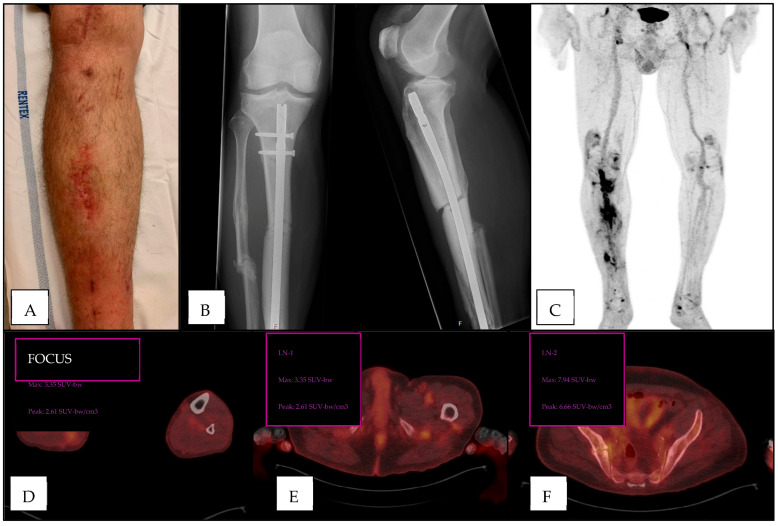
Patient presenting with local erythema and ulceration but no clinical confirmatory signs for FRI, three months after crush injury of the right lower leg with open fracture of the tibia and fibula (A). Plain radiograph with intramedullary nail in situ (B). [18F]FDG-PET/CT showed increased uptake at the fracture site suggestive for infection (C,D). Inguinal (E) and iliac (F) lymph nodes with increased uptake were seen. Intra-operatively obtained deep tissue cultures were positive for *Staphylococcus aureus*.
